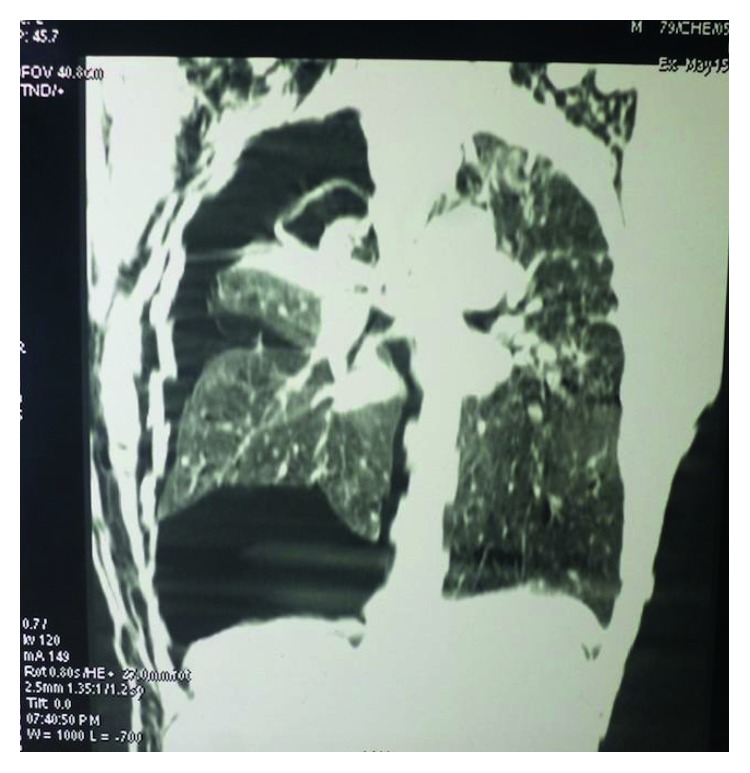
Chest CT scan frontal view. The aspergilloma is seen in the apical region. There is extensive subcutaneous emphysema bilaterally. The upper lobe is totally destroyed, the middle lobe is fibrotic, and the lower lobe is atelectatic and retracted. There are massive pneumothorax, bilateral fibrosis, and sequelae of tuberculosis mainly at the left apex.
